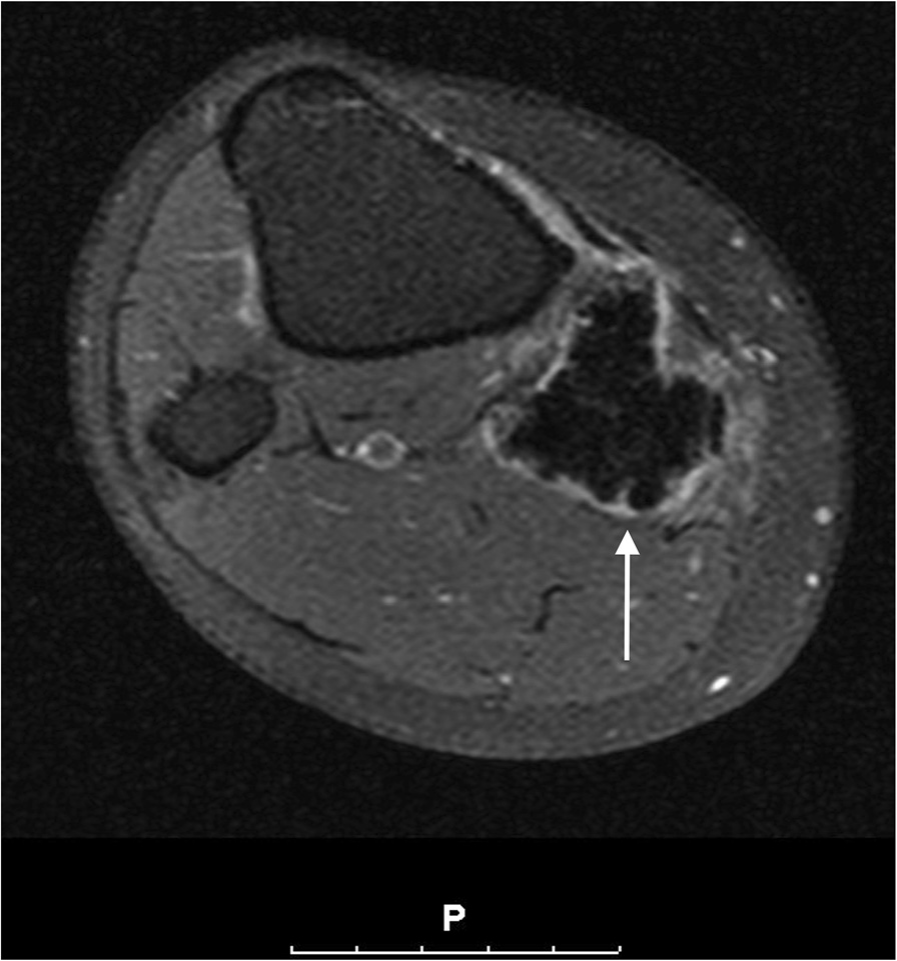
T1-weighted SPIR sequence, transversal cross-sectioning with gadolinium contrast medium enhancement.
